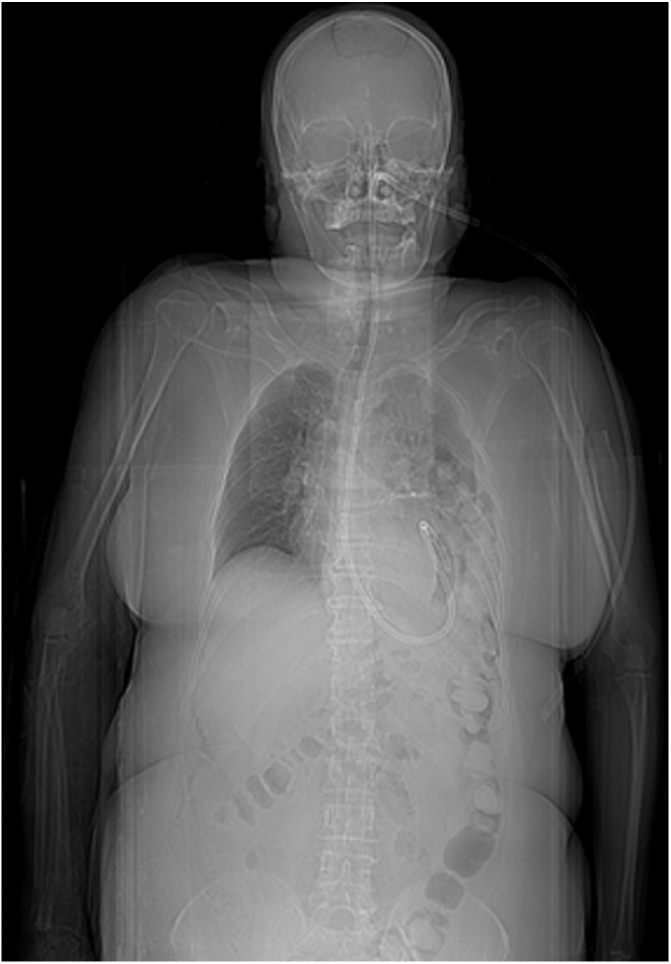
Whole-body non-contrast CT scan (scout view): detection of left-sided posterior diaphragmatic hernia involving transverse colon.
